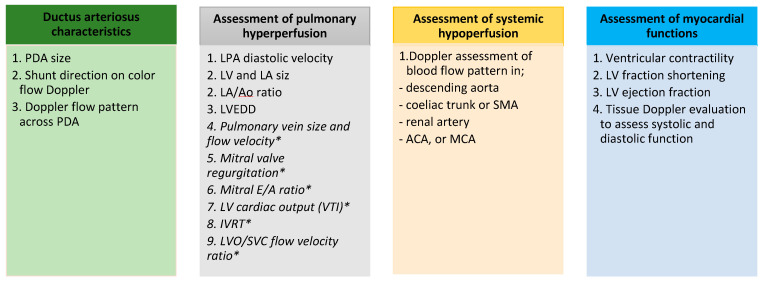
Summary of the echocardiographic assessment of PDA and its hemodynamic impacts. The parameters labeled with * are less commonly used for echocardiographic assessment. *ACA*—*anterior cerebral artery, Ao*—*aorta, Coeliac*—*coeliac trunk, E/A*—*early diastolic flow velocity (E velocity) vs. late diastolic transmitral flow velocity (A velocity), IVC*—*inferior vena cava, IVRT*—*isovolumic relaxation time, MCA*—*posterior cerebral artery, L→R, left-to-right shunt, LA*—*left atrium, LPA*—*left pulmonary artery, LV*—*left ventricle, LVEDD*—*left ventricular end-diastolic diameter, pulmonary artery LVO*—*left ventricular cardiac output, PA*—*pulmonary artery, PDA*—*patent ductus arteriosus, PV*—*pulmonary vein, RA*—*right atrium, RV*—*right ventricle SMA*—*superior mesenteric artery, SVC*—*superior vena cava, VTI*—*velocity time integral*.
